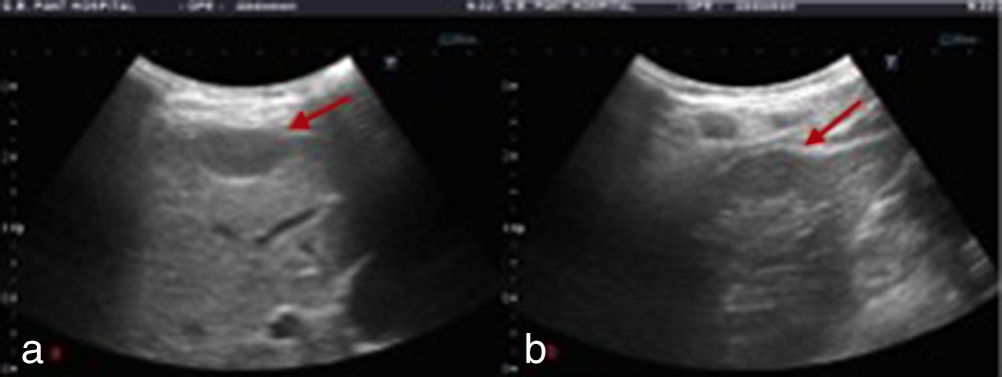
(a, b) Ultrasound images showing hypoechoic, oval-shaped subcapsular hepatic lesions (arrows).
